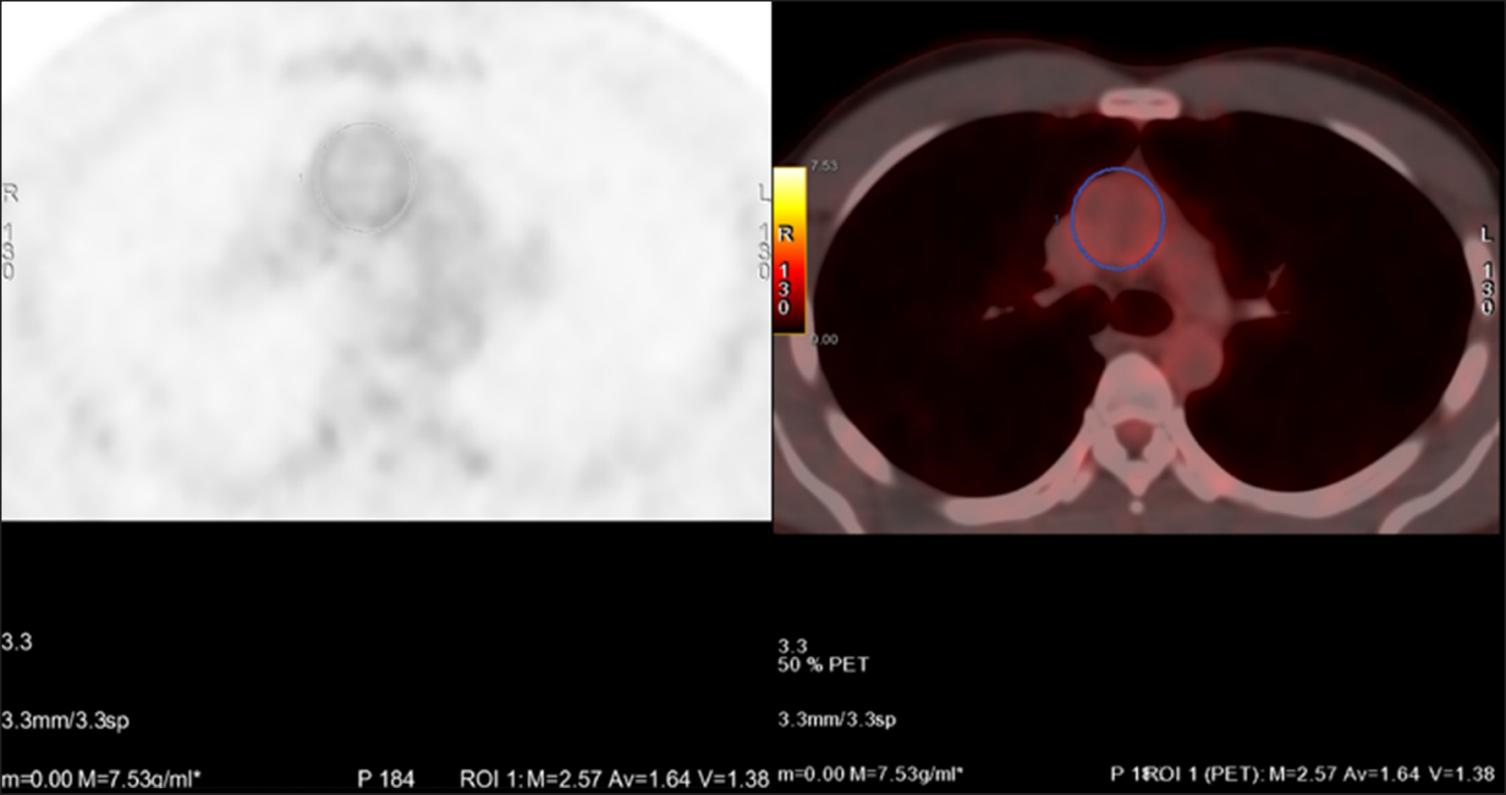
Standard uptake values measurements at various levels of axial slices of the ascending aorta. The SUVmax is depicted as M and SUVmean as Av.
